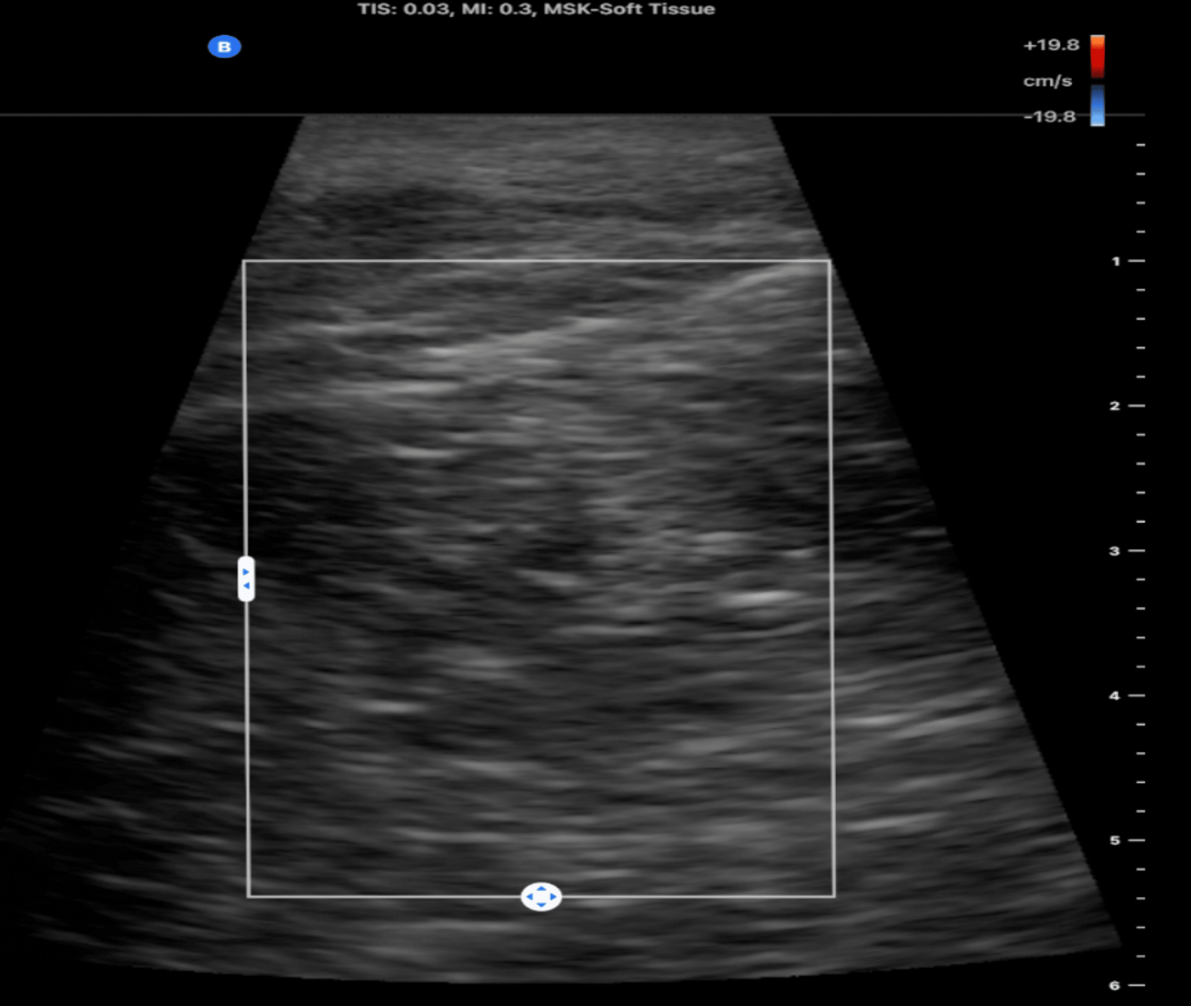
Point-of-care ultrasonographic imaging of the right lateral hip revealed a lipomatous mass.
